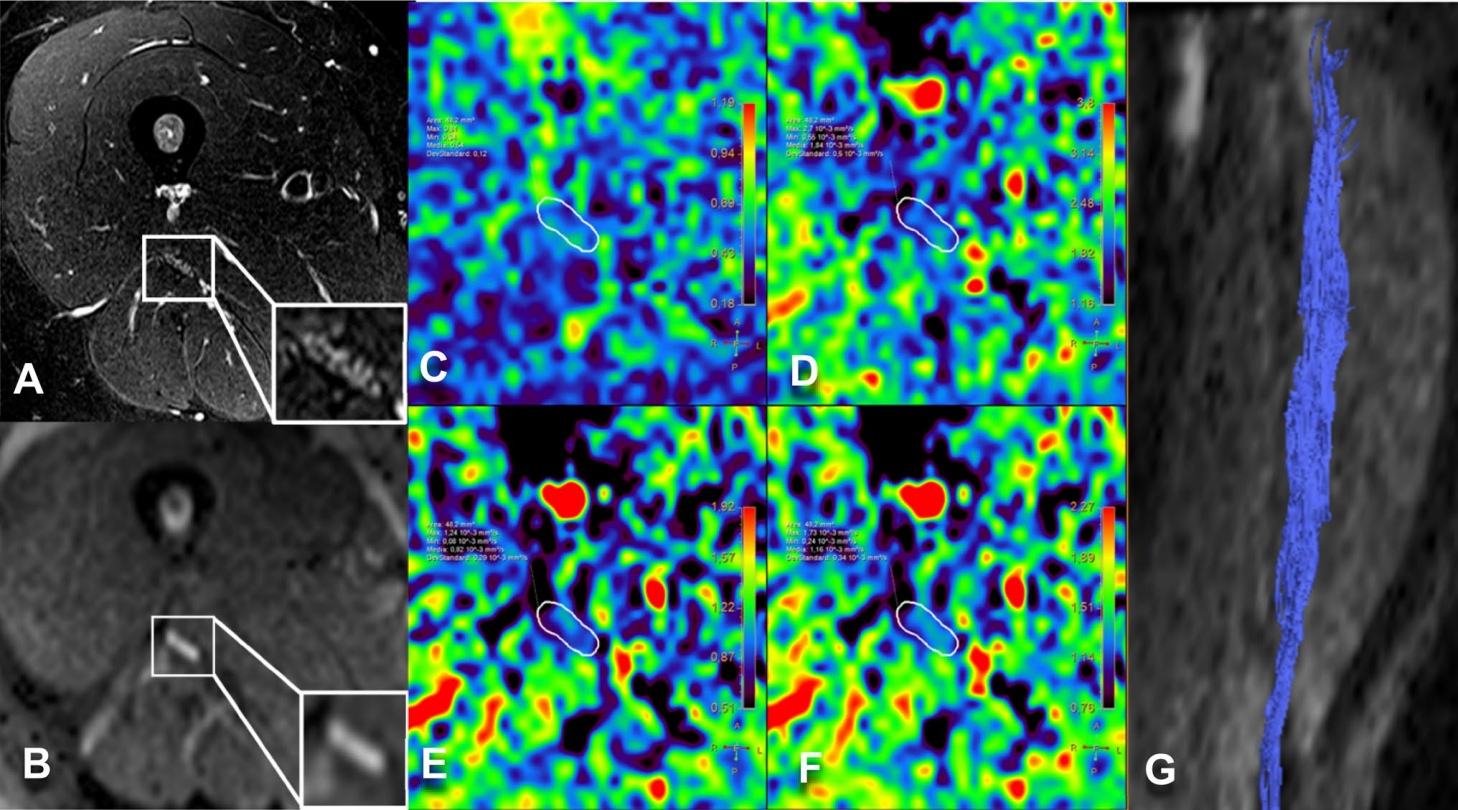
Healthy control. A MR Neurography, sciatic nerve at mid-thigh. B DWI trace-weighted image (*b* = 700), C FA, D AD, E RD, F MD maps, G sciatic nerve tractography at thigh. Sciatic nerve CSA 48 mm^2^, NSI = 1.16, FA = 0.54, AD = 1.84 10^–3^ mm^2^/s, RD = 0.82 10^–3^ mm^2^/s, ADC = 1.16 10^–3^ mm^2^/s
